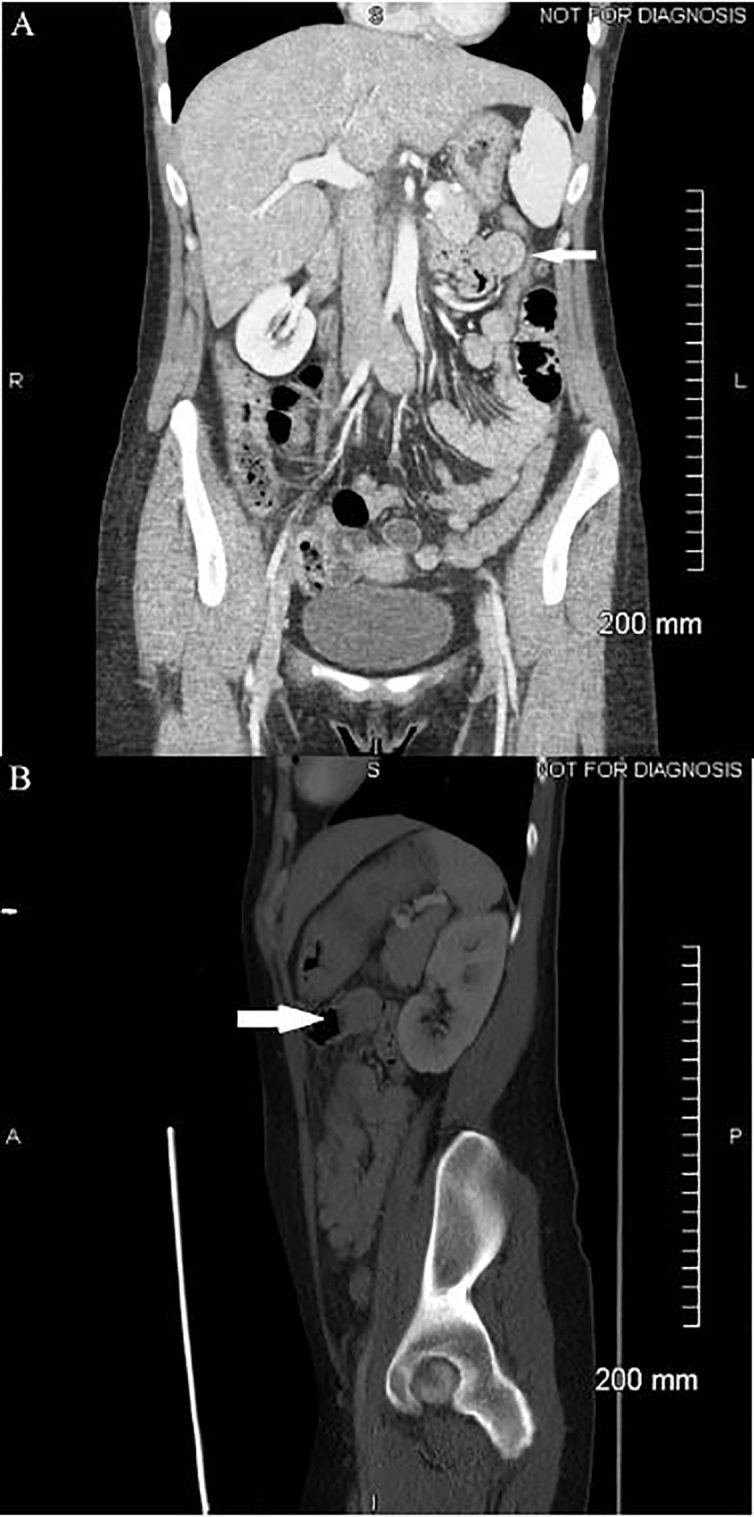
Computed tomography (CT) images of the abdomen and pelvis demonstrating transient, non-obstructive jejunal intussusception: (A) Coronal view depicting the classic “target pattern” (small arrow) in the left upper quadrant; (B) Saggital view showing the jejunal short segment intussusception (big arrow) without any evidence of bowel obstruction.
